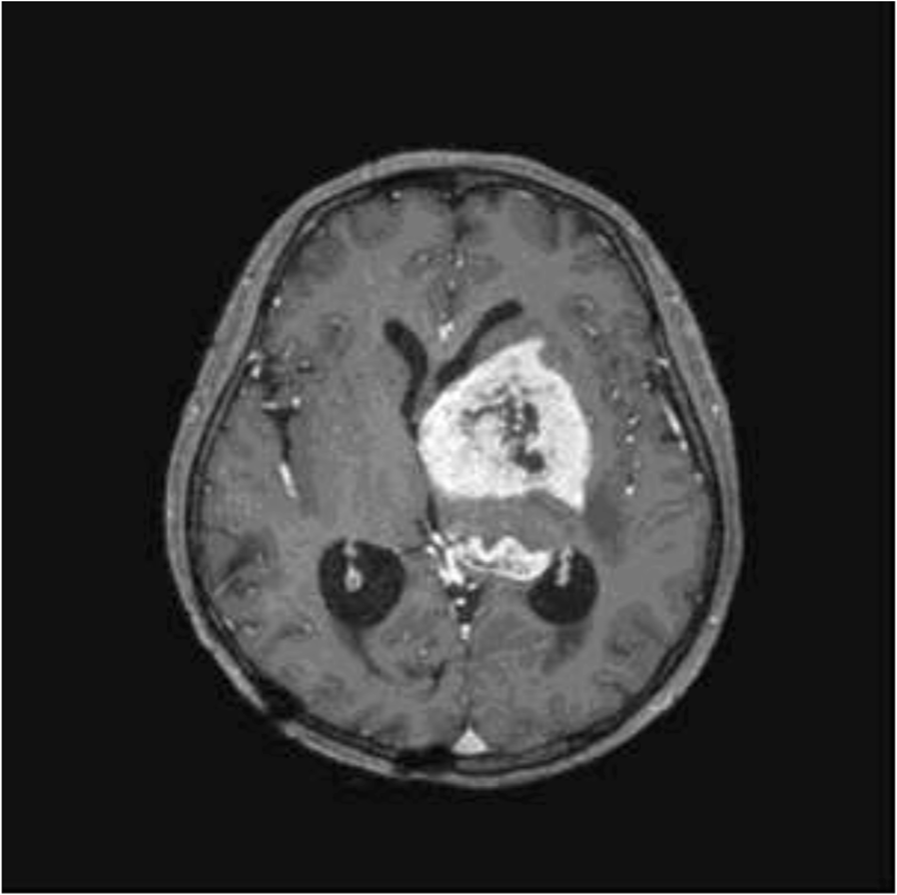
MRI demonstrated a tumor as a gadolinium-enhanced lesion in the left basal ganglia with extension to crus cerebri and thalamus
